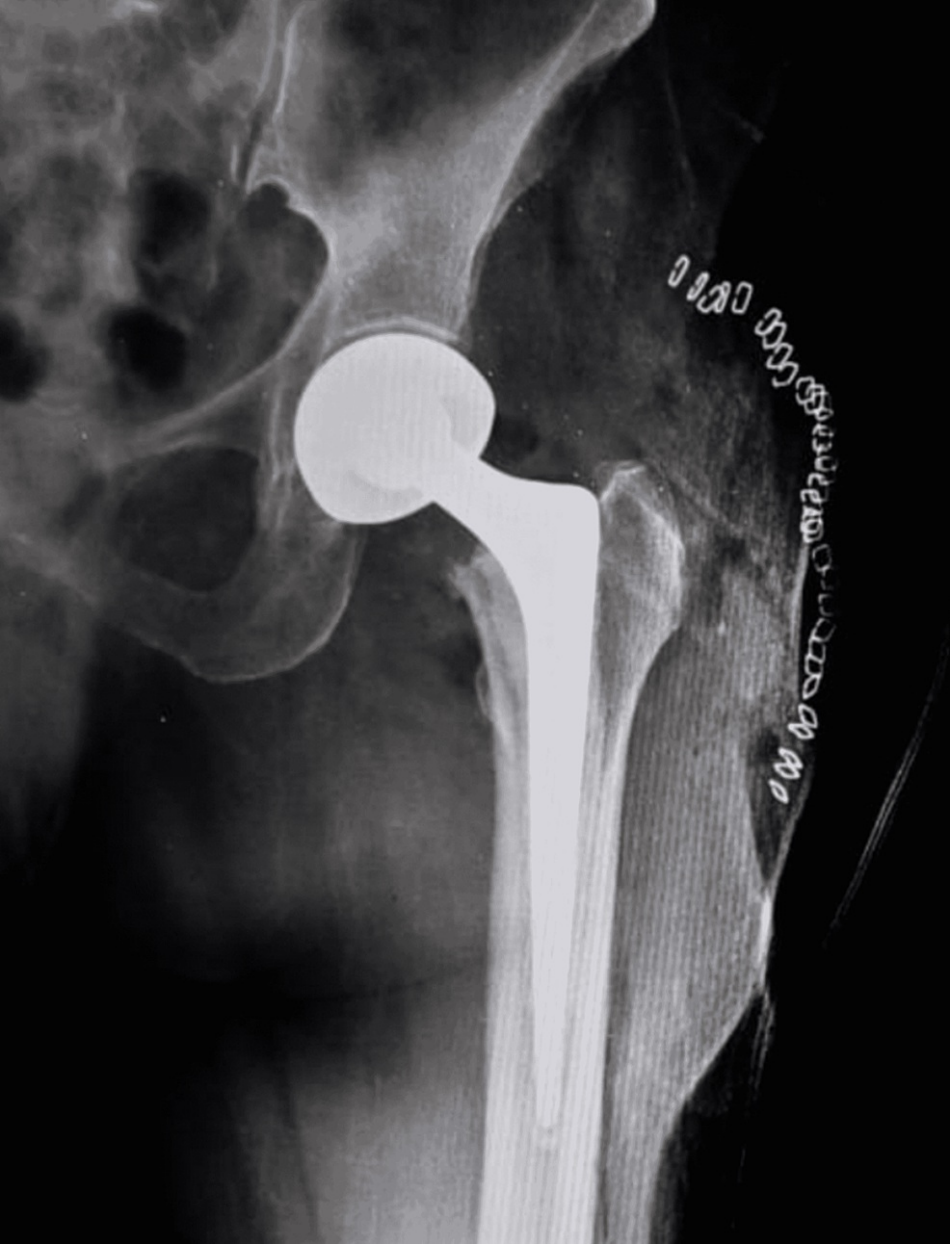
Postoperative X-ray showing bipolar hemiarthroplasty X-ray showing a well-fixed implant. The version appears to be within normal limits.
